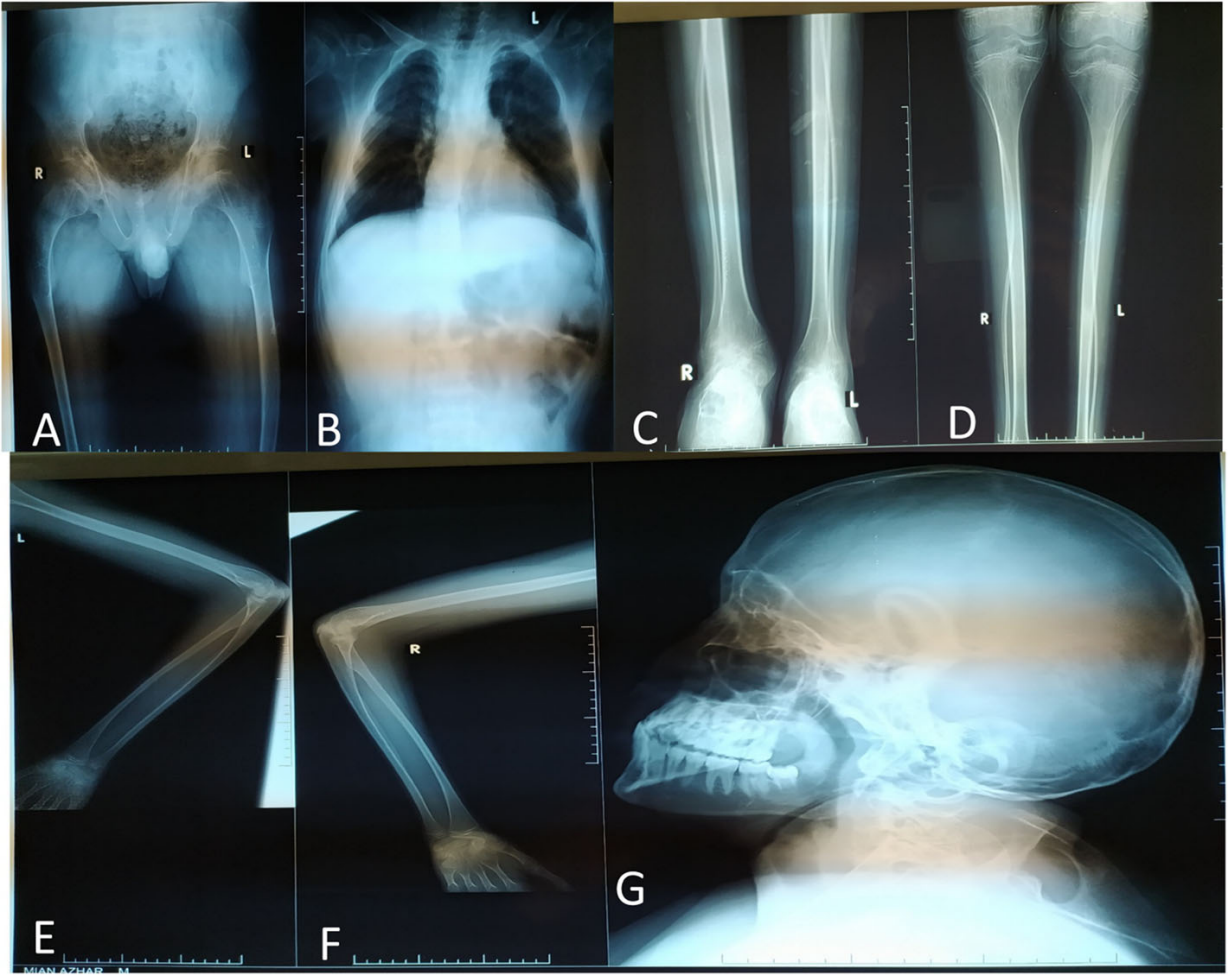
Radiographic findings in Patient VI:3. a. Frontal radiograph of the pelvis shows increased bone density, trabecular thickening and ossification of the sacrotuberous ligament. Mild flattening of the acetabular roof is noted giving rise to champagne glass deformity of the pelvis. b Both lung fields are clear Bilateral CP angles are sharp. Mediastinal contours appear unremarkable. Scoliotic deformity of the spine is noted with convexity towards right side. c Outward bowing of bilateral femur is noted. d Bilateral fibula shows marked thinning and outward bowing (e, f) outward bowing of bilateral humerus bone is also noted. g Long oval face hypoplasia and bone deformities
